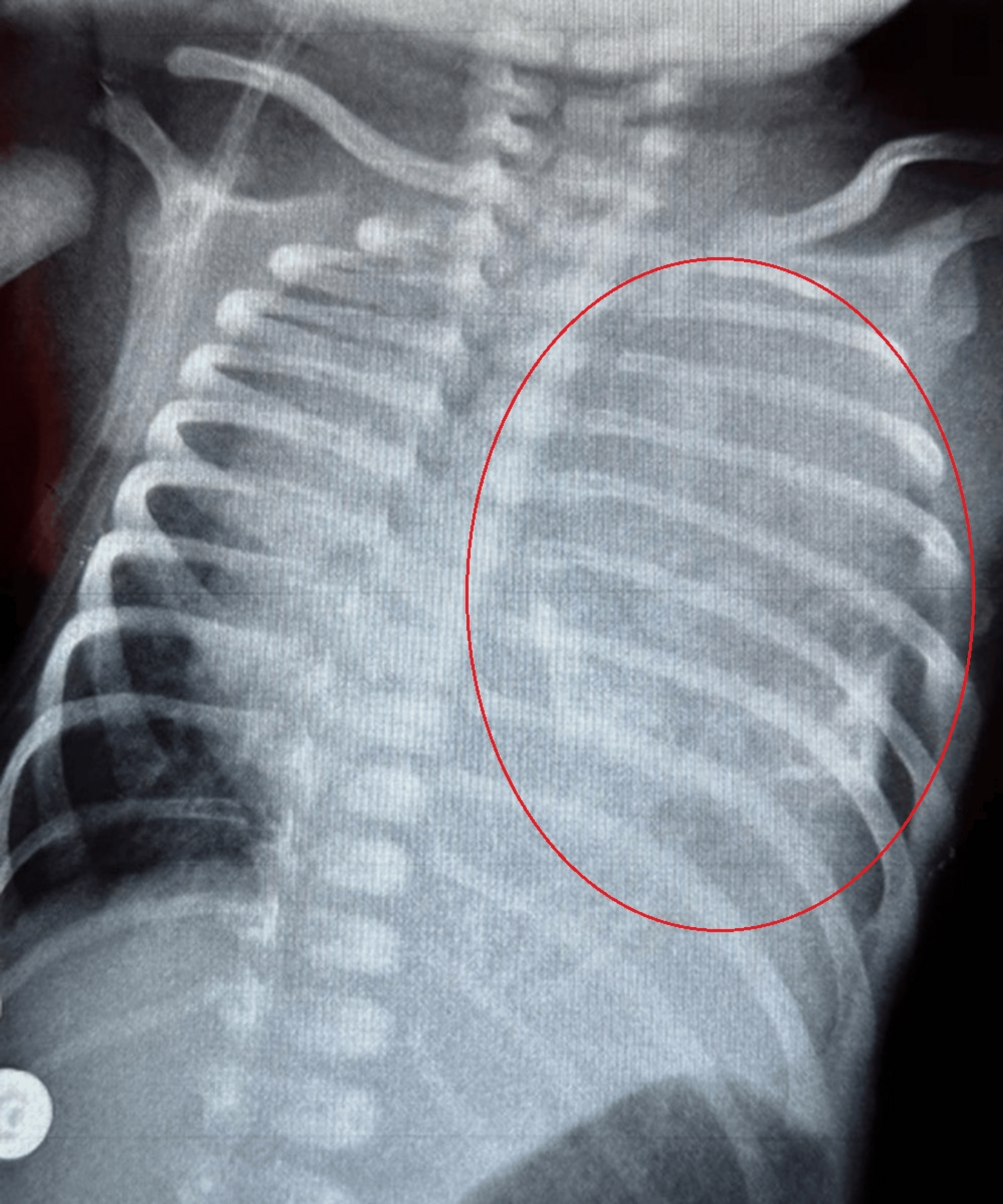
Chest X-ray showing a left-side intrathoracic mass The red circle shows the mass in the left hemithorax.
